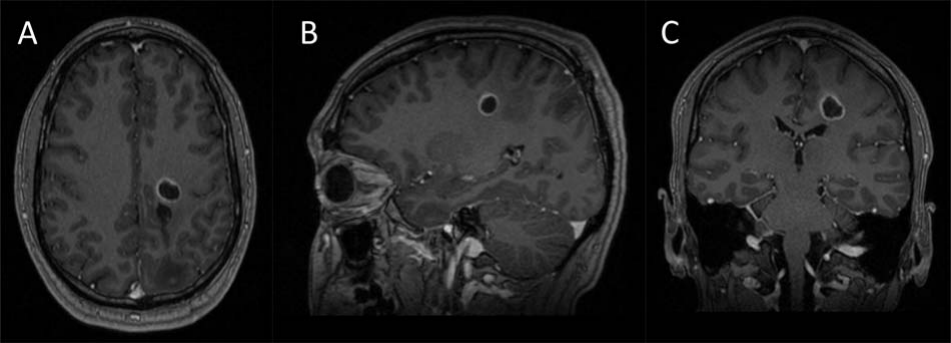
Preoperative imaging; (A–C) axial, sagittal and coronal T1-wieghted magnetic resonance imaging (MRI) images, with gadolinium demonstrating a contrast enhancing lesion in the left corona radiata.
